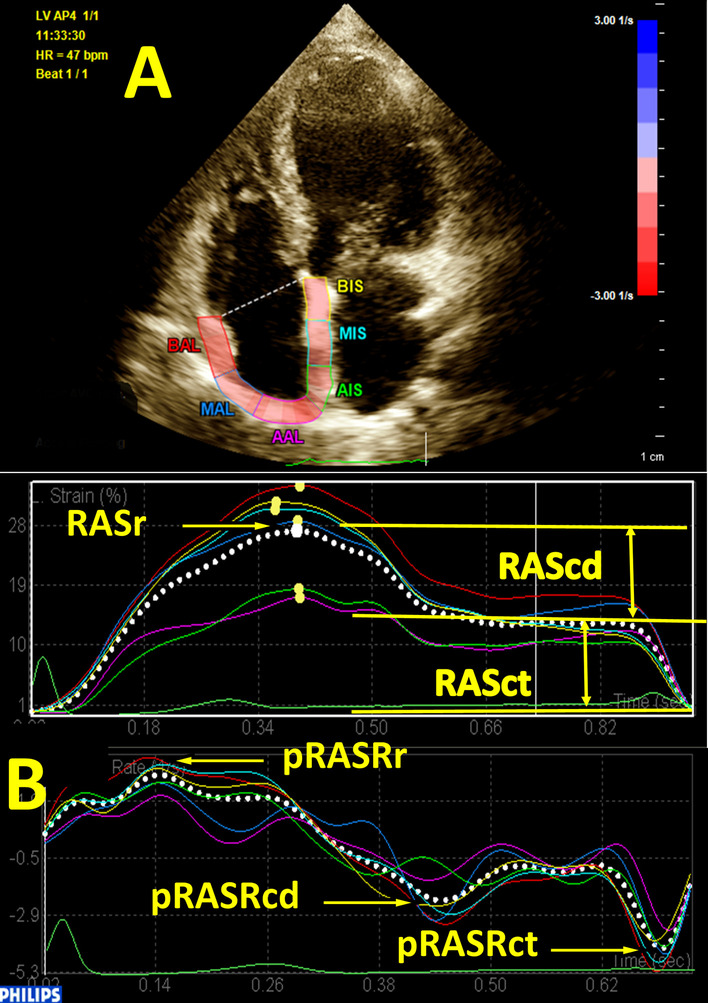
The image depicts 2D speckle-tracking echocardiography of the right atrium in the modified apical 4-chamber view. A strain curves, B strain rate curves. pRASRcd, peak right atrial longitudinal strain rate during the conduit phase; *pRASRct* peak right atrial longitudinal strain rate during the contraction phase, *pRASRr* peak right atrial longitudinal strain rate during the reservoir phase, *RAScd* right atrial longitudinal strain during the conduit phase, *RASct* right atrial longitudinal strain during the contraction phase, *RASr* right atrial longitudinal strain during the reservoir phase
